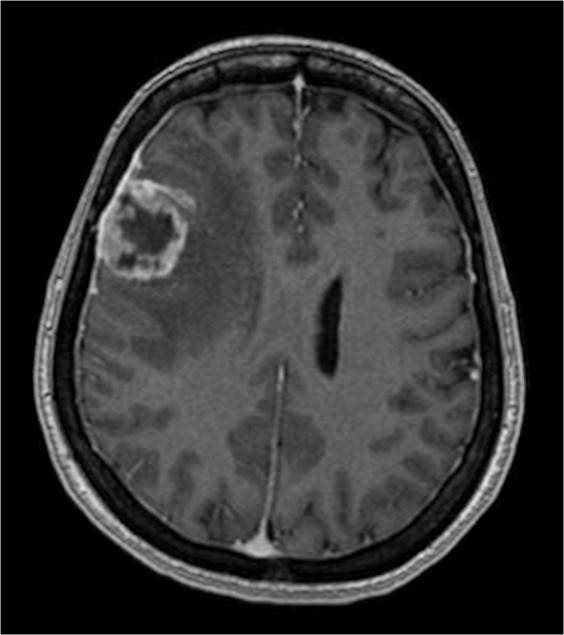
T1-weighted gadolinium-enhanced axial MRI brain demonstrating a low complexity lesion with a 74.9% likelihood of complete resection.
